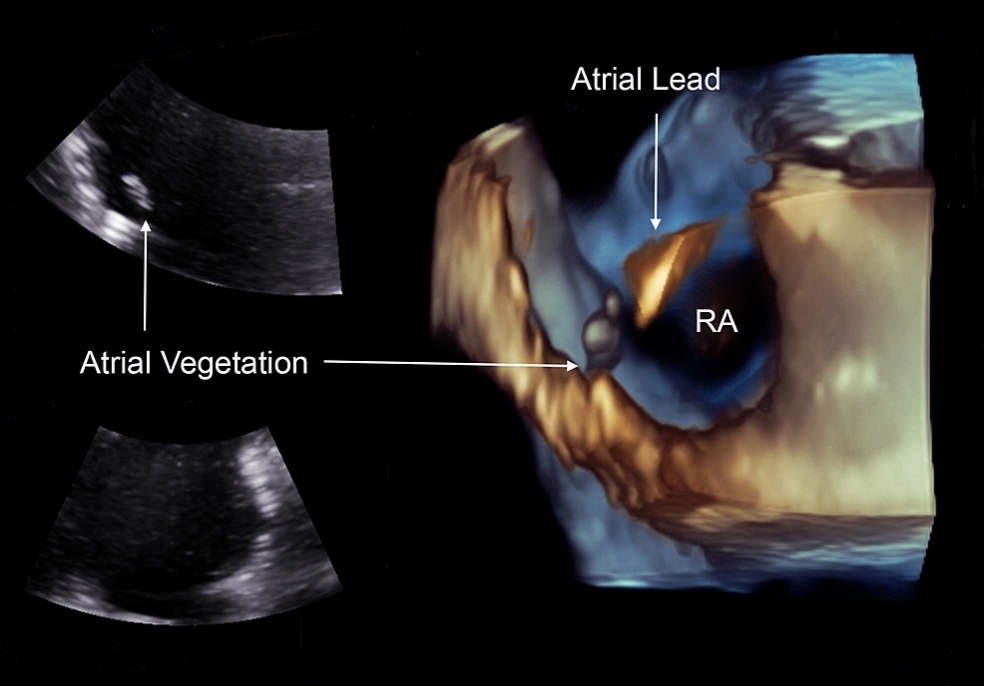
TEE showing a 0.42cm x 1.92cm vegetation attached to the right atrial lead RA - right atrium; TEE - transesophageal echocardiogram
